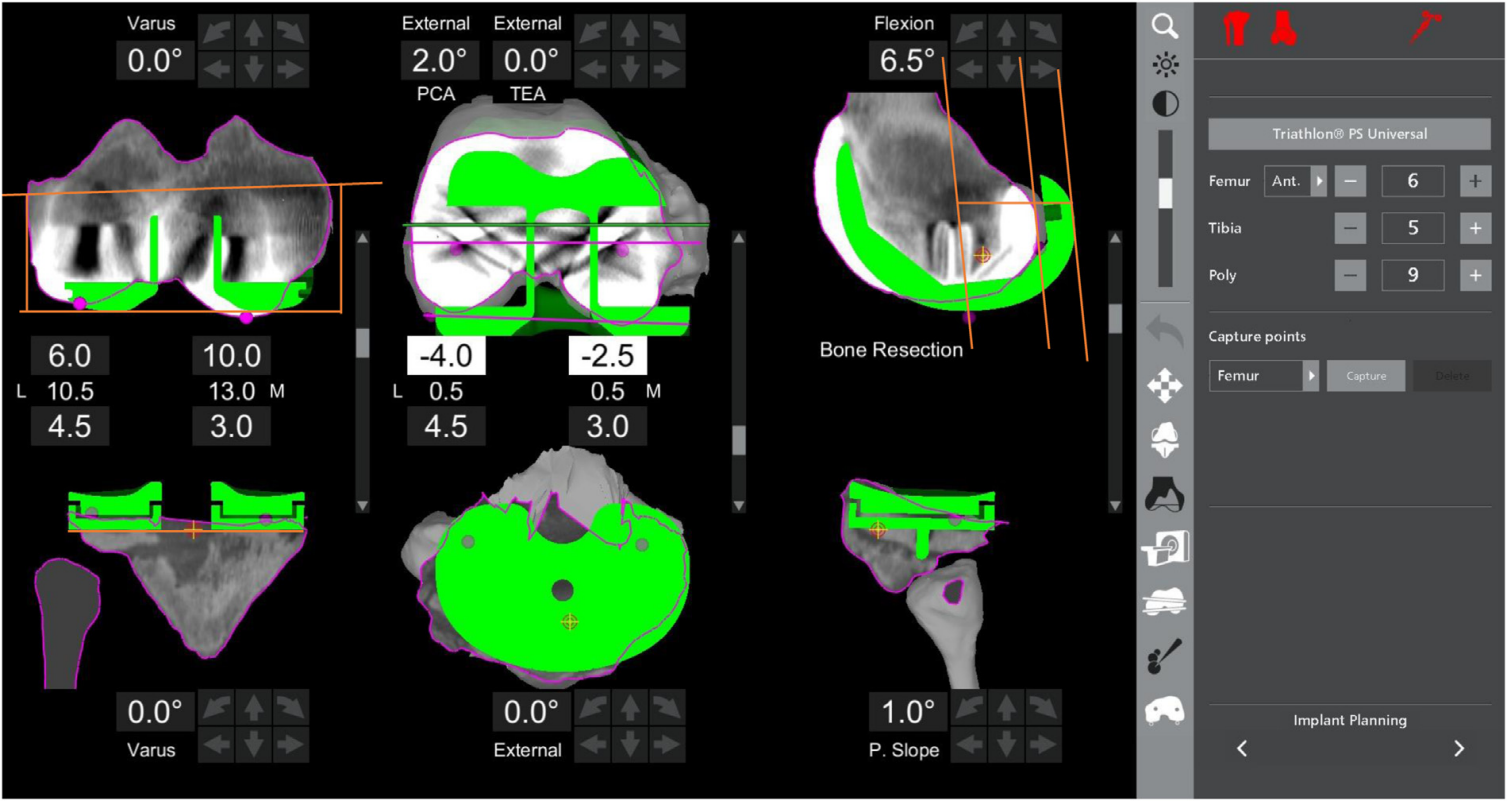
Robotic implant planning page depicting the final position of the robotic revision implant (RRI) in green overlying the Primary implants (PI) in white. Top right: Note the increased posterior offset between the RRI and PI necessary to balance the revision TKA. Metal artifact can obscure bony landmarks, but by viewing multiple slices of the CT scan, we can best estimate the location of the bony landmarks. Because revision TKA is not yet indicated for the MAKO robotic system, revision implants and intramedullary stems are not currently available for virtual templating.
